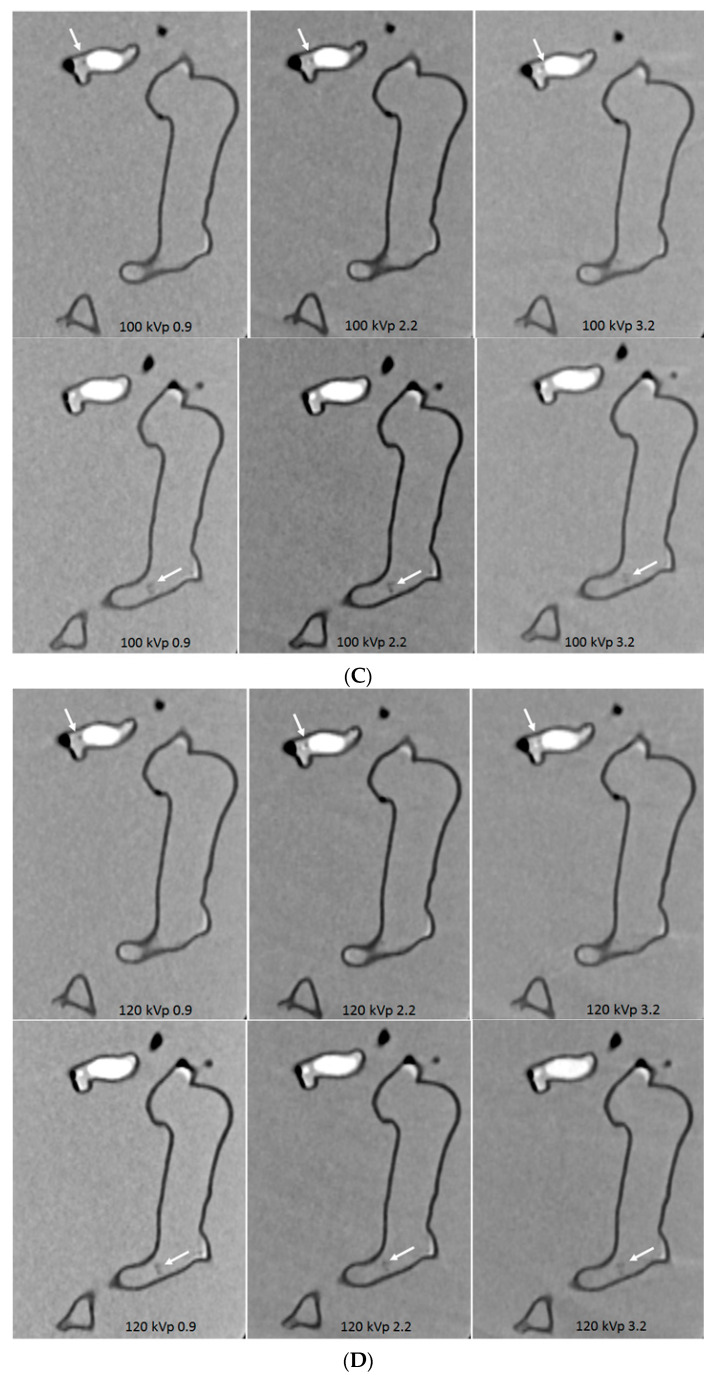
Computed tomography pulmonary angiography (CTPA) in the detection of peripheral pulmonary embolism with different scanning protocols. (A–D) The small thrombus is viewed as low-attenuation filling defect in the left segmental pulmonary artery (top arrows) and right pulmonary artery (bottom arrows) and they are visible in all protocols, regardless of kVp or pitch values used. Reprinted with permission under open access from Aldosari et al. [84].
